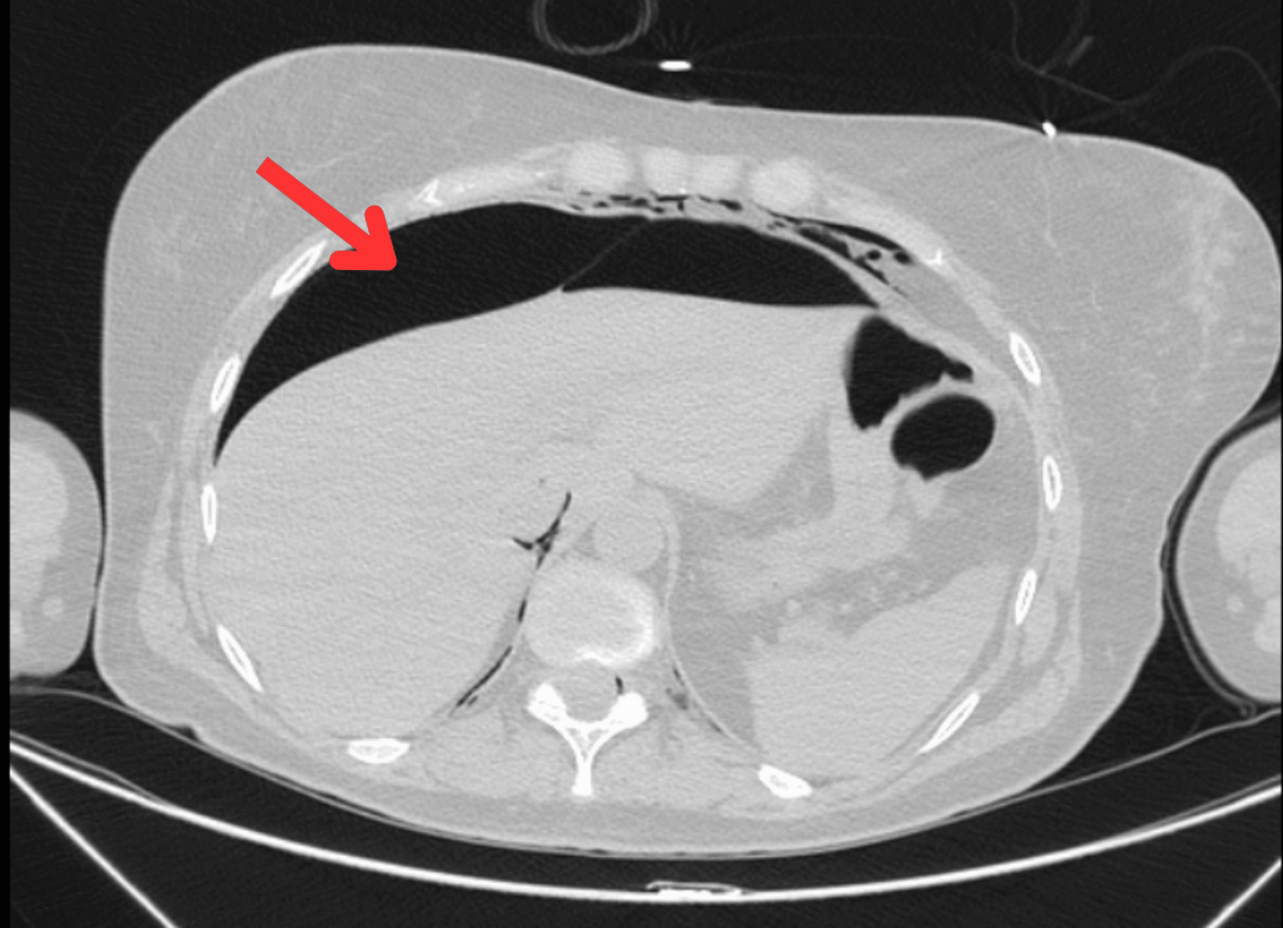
CT image (axial): pneumoperitoneum (arrow).
